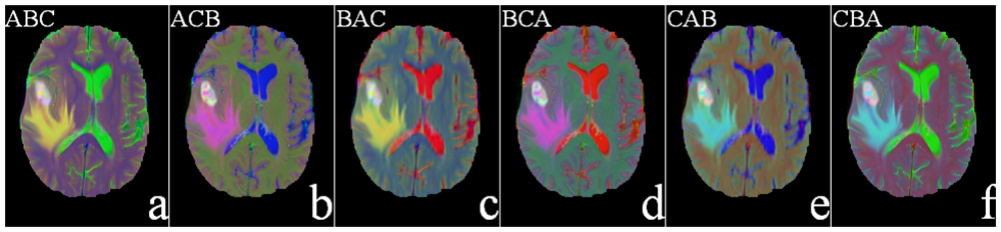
Six pseudo-colored brain MR sequences. (a) *FLAIR*—*T*2—*T*1*C*, (b) *FLAIR*—*T*1*C*—*T*2 (c) *T*2—*FLAIR*—*T*1*C*(d) *T*2—*T*1*C*—*FLAIR*(e) *T*1*C*—*FLAIR*—*T*2, and (f) *T*1*C*—*T*2—*FLAIR*, as mapped to the *RGB* plane.
